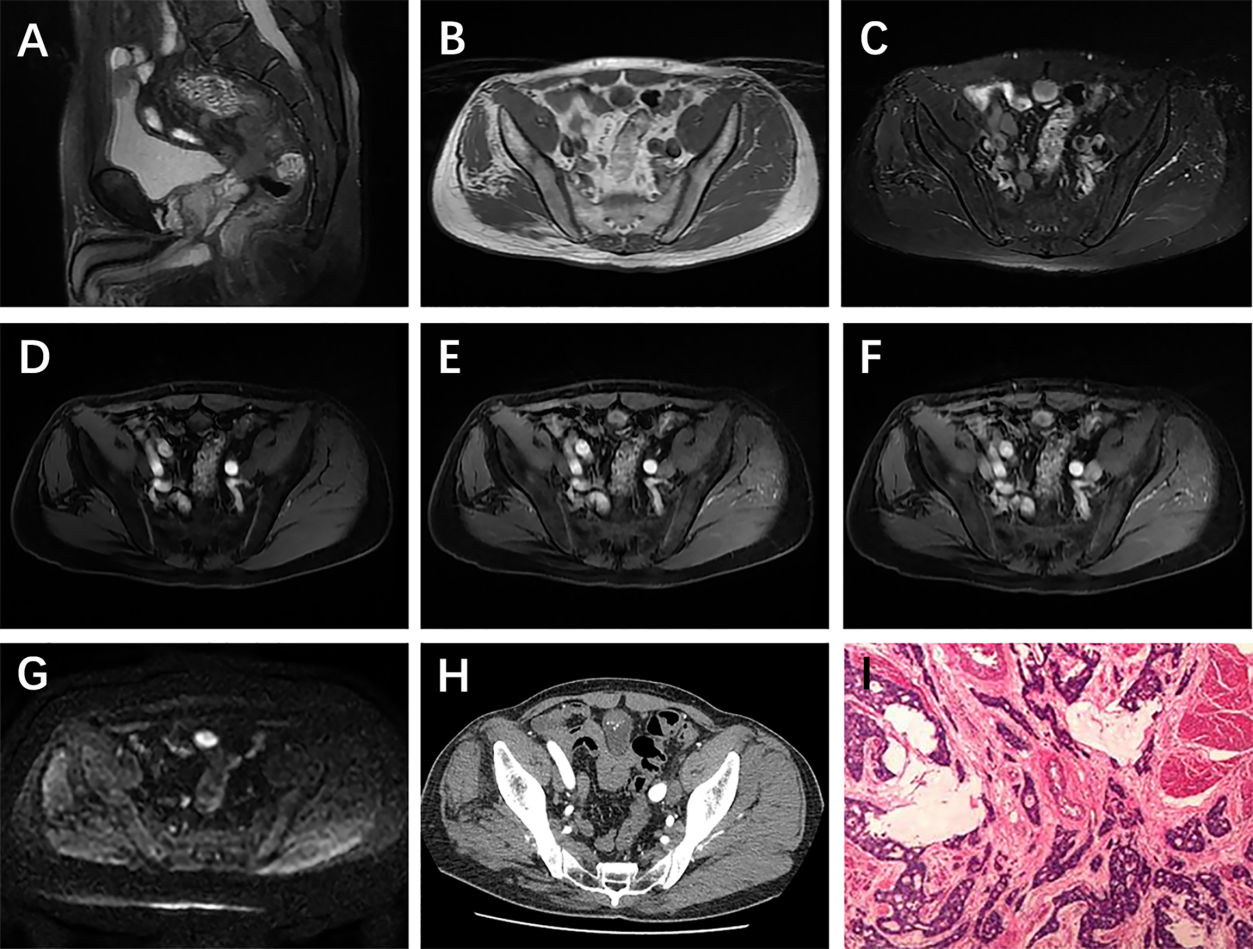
A 62-year-old man with urachal carcinoma presented with painless gross hematuria. Sagittal T2WI (A) showed a solid mass in the urachus; slightly low signal intensity on T1WI (B) and high signal intensity on T2WI (C); axial dynamic contrast-enhanced LAVA-Flex images showed no obvious enhancement in arterial phase, but obvious enhancement in venous phase and delayed phase (D–F); restricted diffusion on DWI (G); multiple spotted calcifications on axial unenhanced CT scan (H); pathological findings showed moderately differentiated adenocarcinoma (intestinal type) (I).
